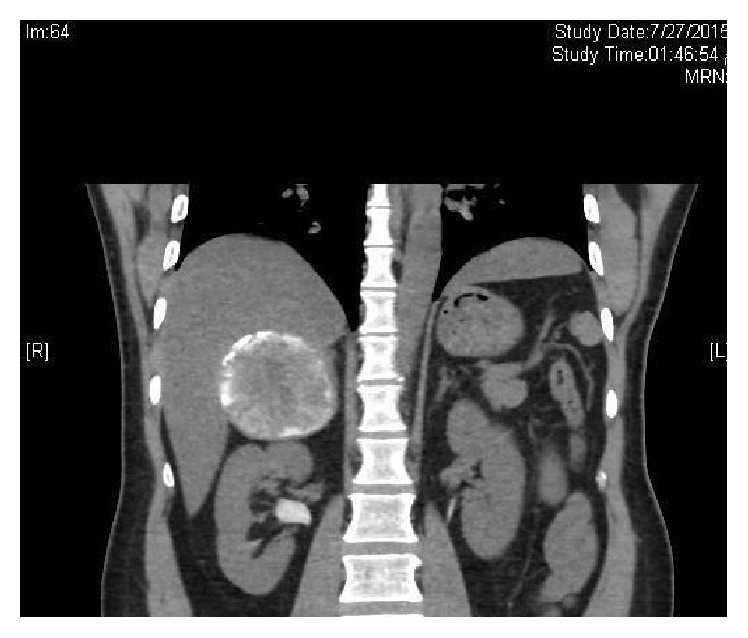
CT scan showing (R) adrenal mass with calcification.
